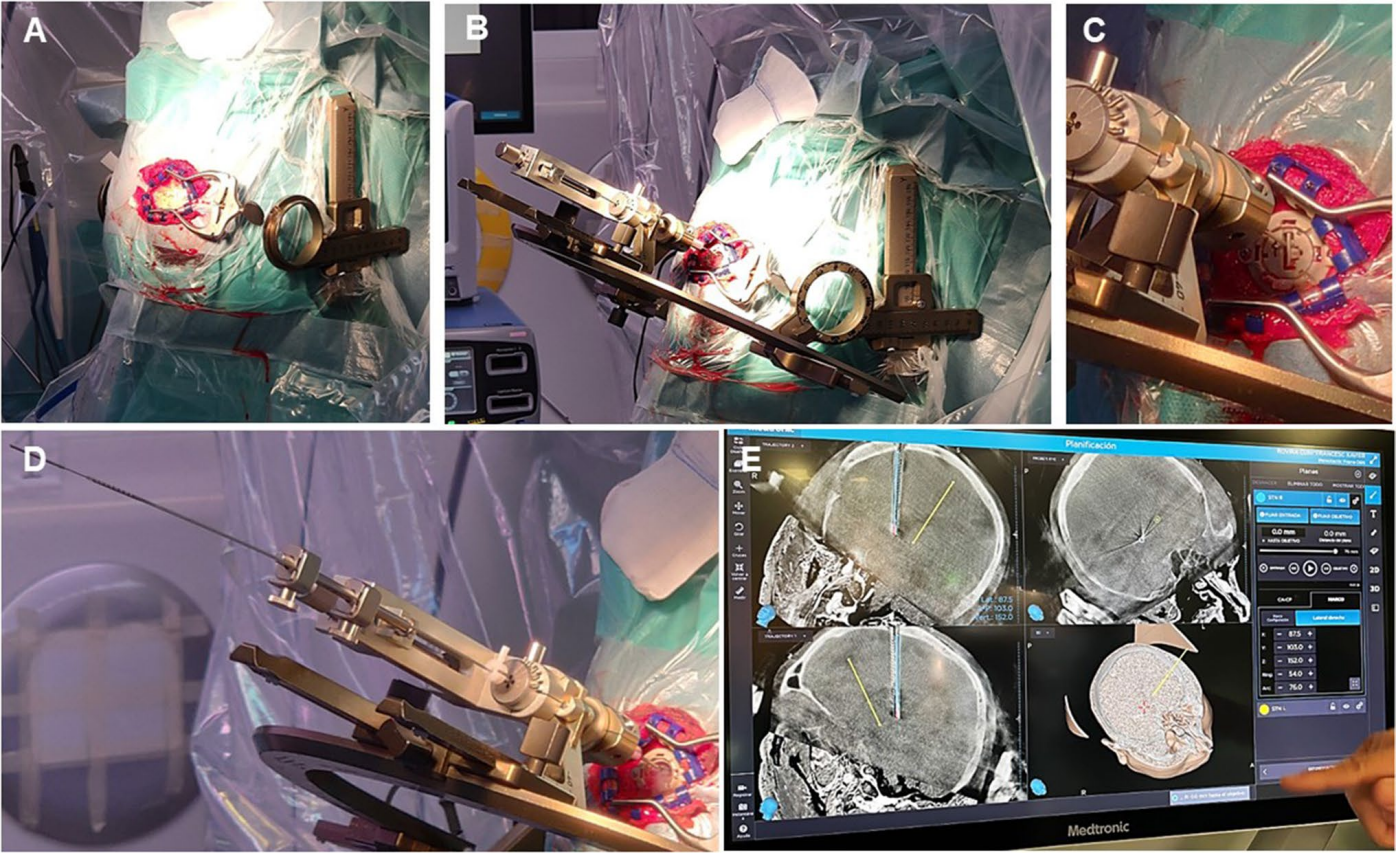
Electrode placement. A 3-cm linear incision is made over the marked point of entry. B The point of the trepanation is indicated with the aid of the frame. C The fixation system is anchored to the burr hole and the dura is opened in a cruciate fashion. Cautious opening of the arachnoid and cerebral cortex will avoid catheter deviation during the introduction. Once the canula is in place, a tissue sealer is applied on the burr hole to prevent CSF outflow and pneumocephalus. D The directional electrode is placed within the canula, with the appropriate orientation, and the canula is removed. F) A second CT is obtained and fused with the preoperative planification to check for the accuracy of the target. If satisfactory, the lock is laid, and the distal portion of the catheter, protected and tunnelled towards the parietal eminence
